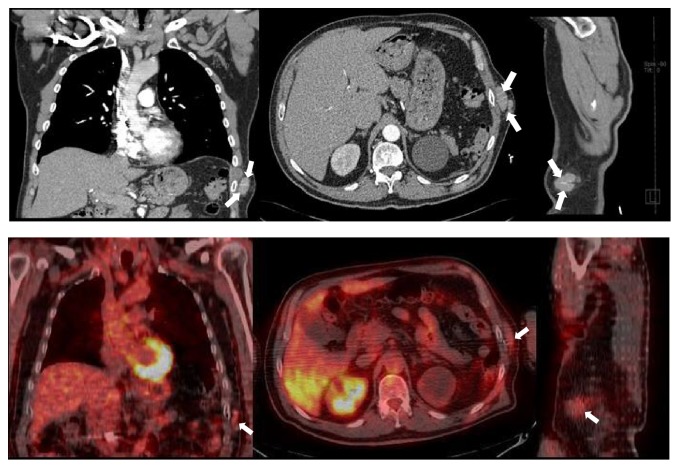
Top row (from left to right): coronal, axial, and sagittal CT Chest with IV contrast soft tissue views highlighting the left chest wall subcutaneous lesions. Bottom row (from left to right): coronal, axial, and sagittal fused PET/CT showing FDG uptake to the same lesions.
